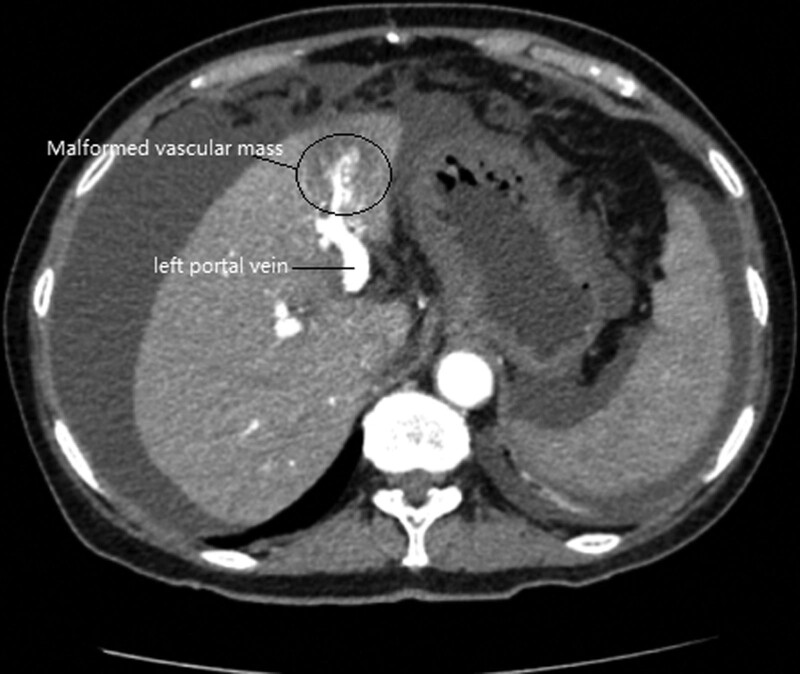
Contrast-enhanced CT scan: An area of abnormal low-density changes with an ambiguous boundary was evident in the S4 segment of the liver. The portal vein was highlighted in the arterial phase, and widening of the left branch of the portal vein was evident. CT = computed tomography.
